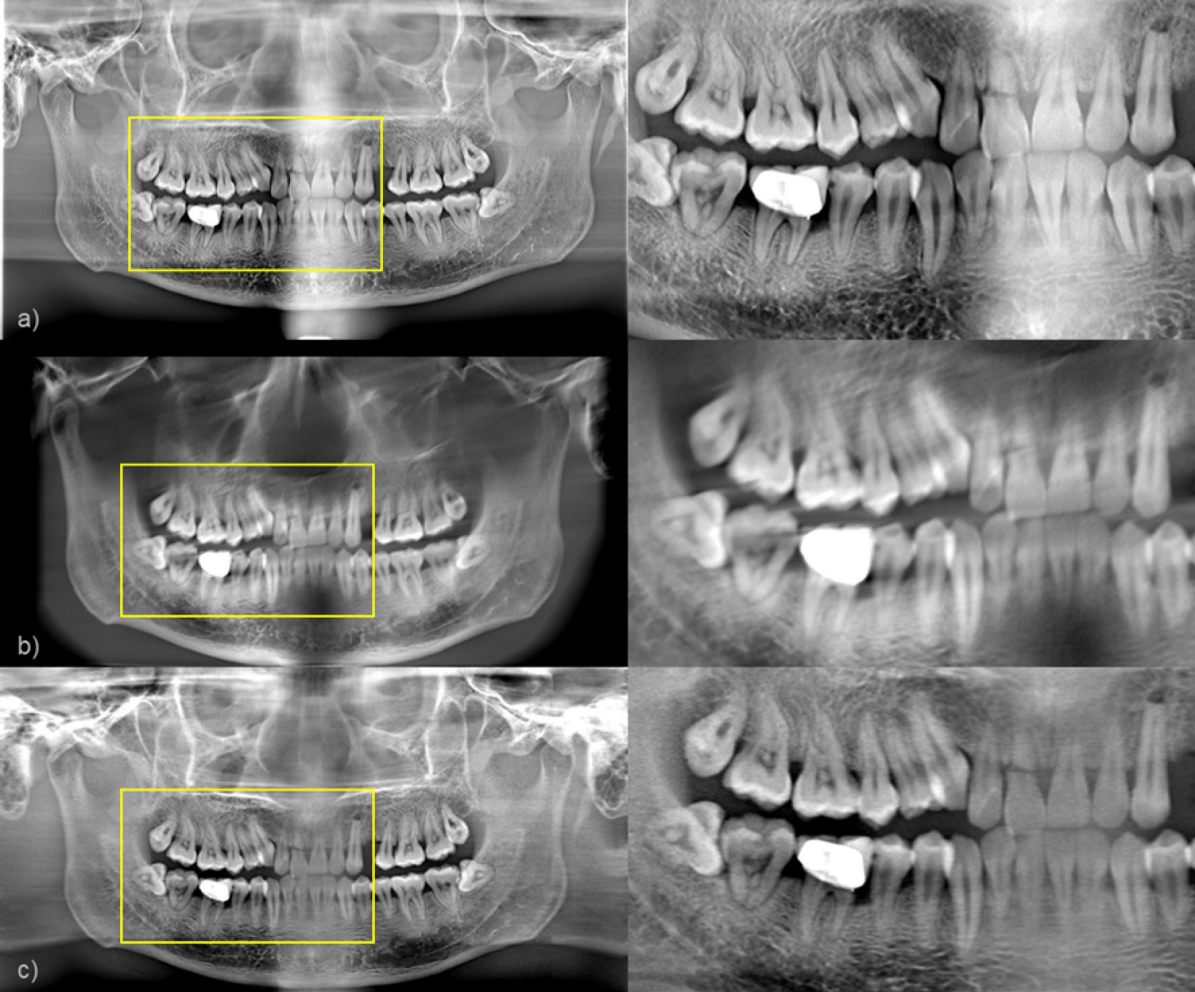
(a) A panoramic image obtained by the panoramic scan system, (b) A panoramic image synthesized from the dental CBCT image, and (c) A panoramic image obtained by the proposed method.
